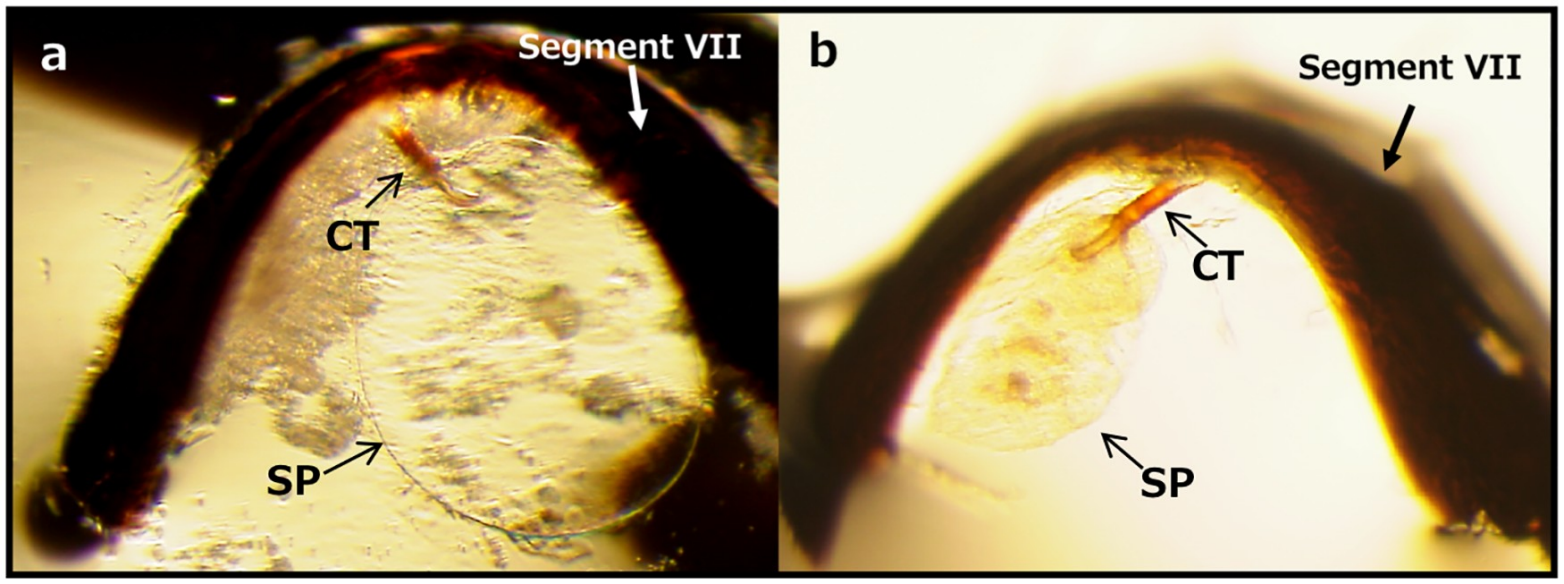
Copulatory tube with sperm pouch. A copulatory tube and sperm pouch isolated from a virgin female (a) and mated female (b) of *O*. *strigicollis*. The copulatory tube was connected to abdominal segment VII via an intersegmental membrane. CT, copulatory tube; SP, sperm pouch.
